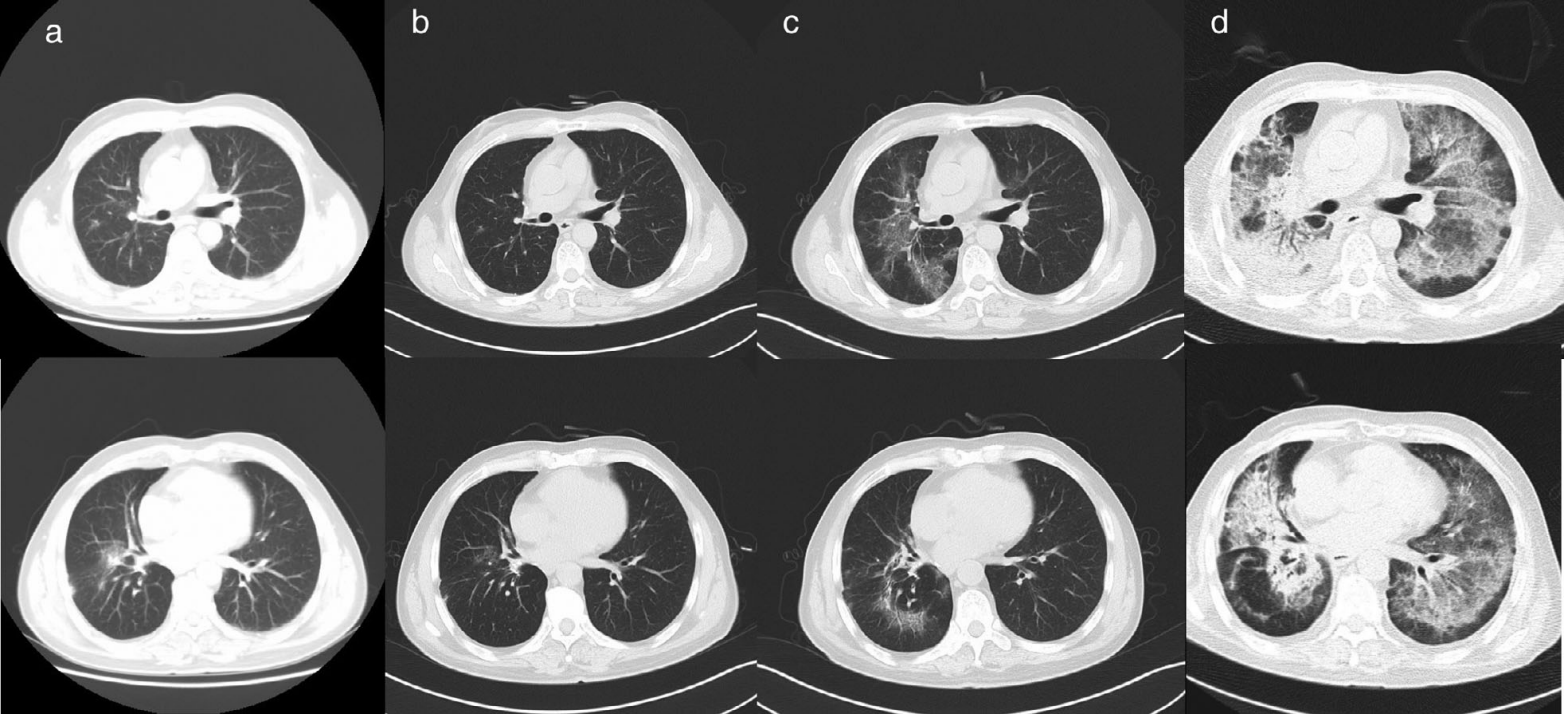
Computed tomography (CT) scan findings in Case 3. (a) Multiple irregular opacities on the right lung and radiation pneumonia were suspected. (b) There was remission of radiation pneumonia after steroid treatment. (c) Ground‐glass opacities (GGOs) were evident when the patient developed fever. (d) There were extensive ground‐glass opacities (GGOs) and multifocal consolidations bilaterally.
